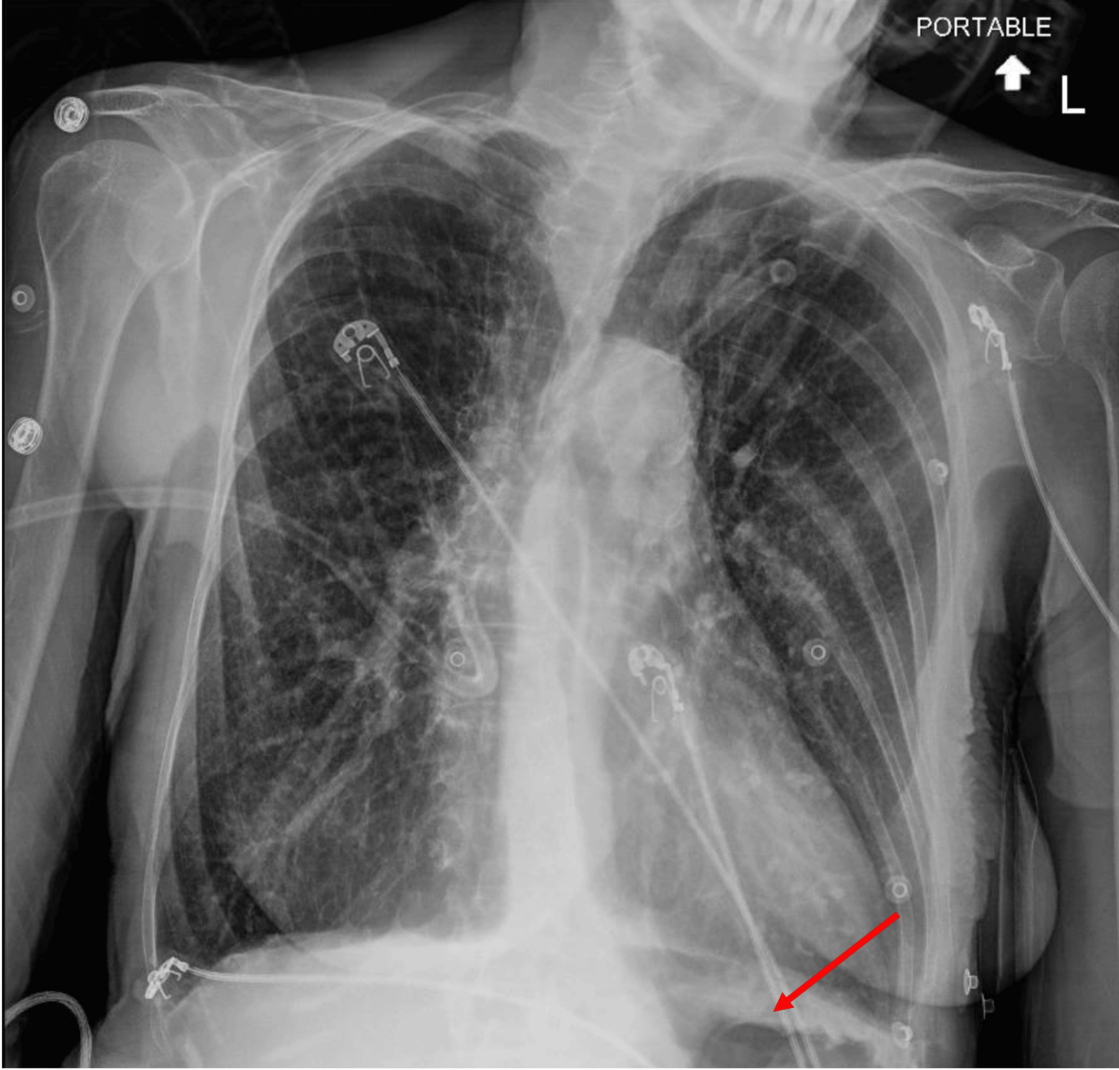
Chest radiograph demonstrating air under left diaphragm (red arrow) and bilateral interstitial opacities.
